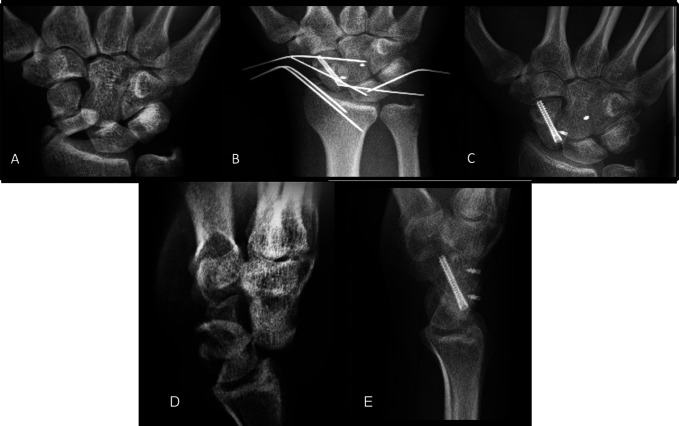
Case 1 serial right wrist, navicular view radiographs demonstrating (A) 1-day post-trauma, (B) postoperative, and (C) 22 weeks postoperative. Lateral wrist radiographs (D) at 1 day and (E) 22 weeks post-trauma.
